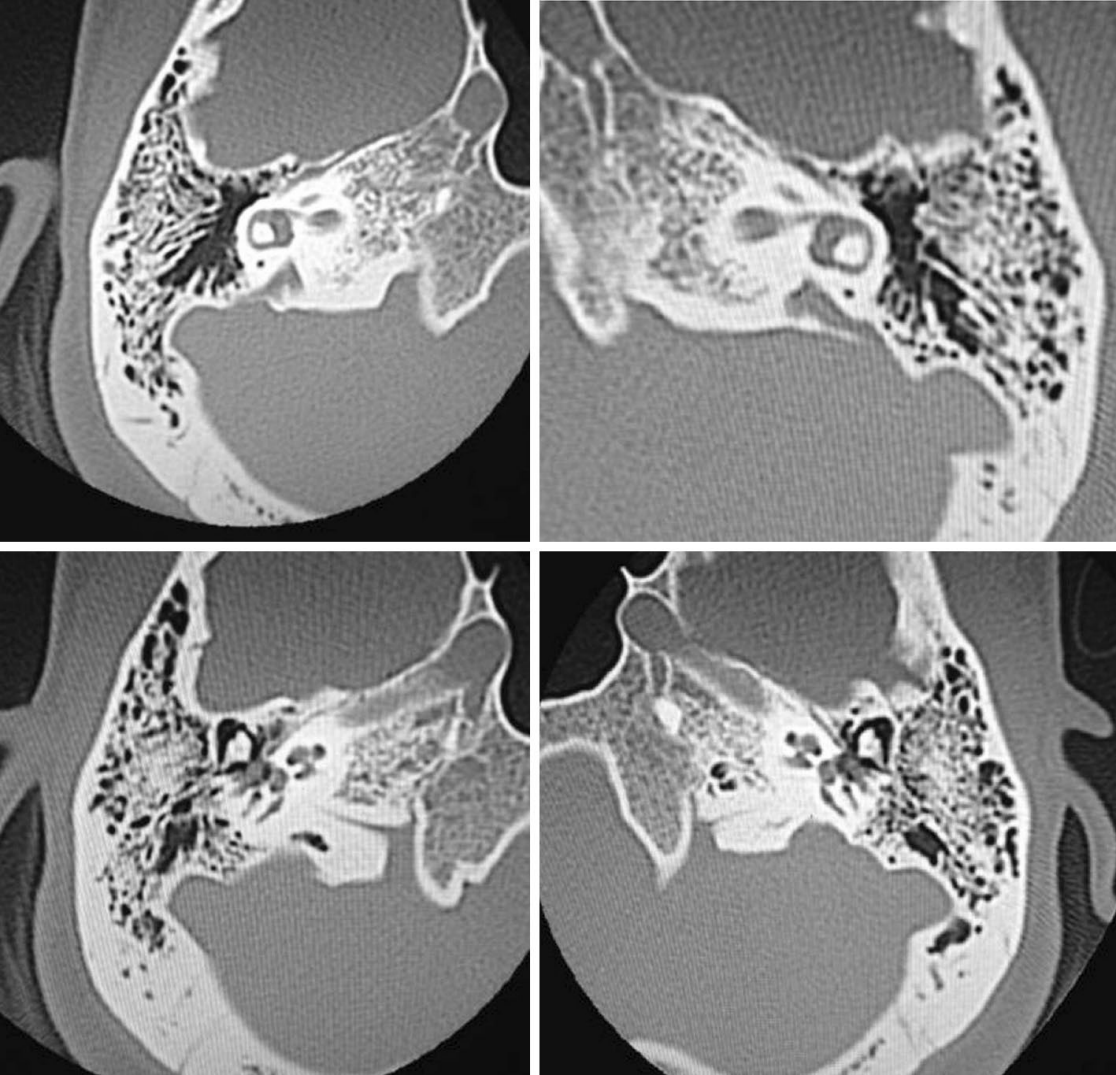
CT scan, axial section, there is an enlarged vestibular aqueduct and malformation of the cochlea, corresponding to Mondini dysplasia in both sides.
